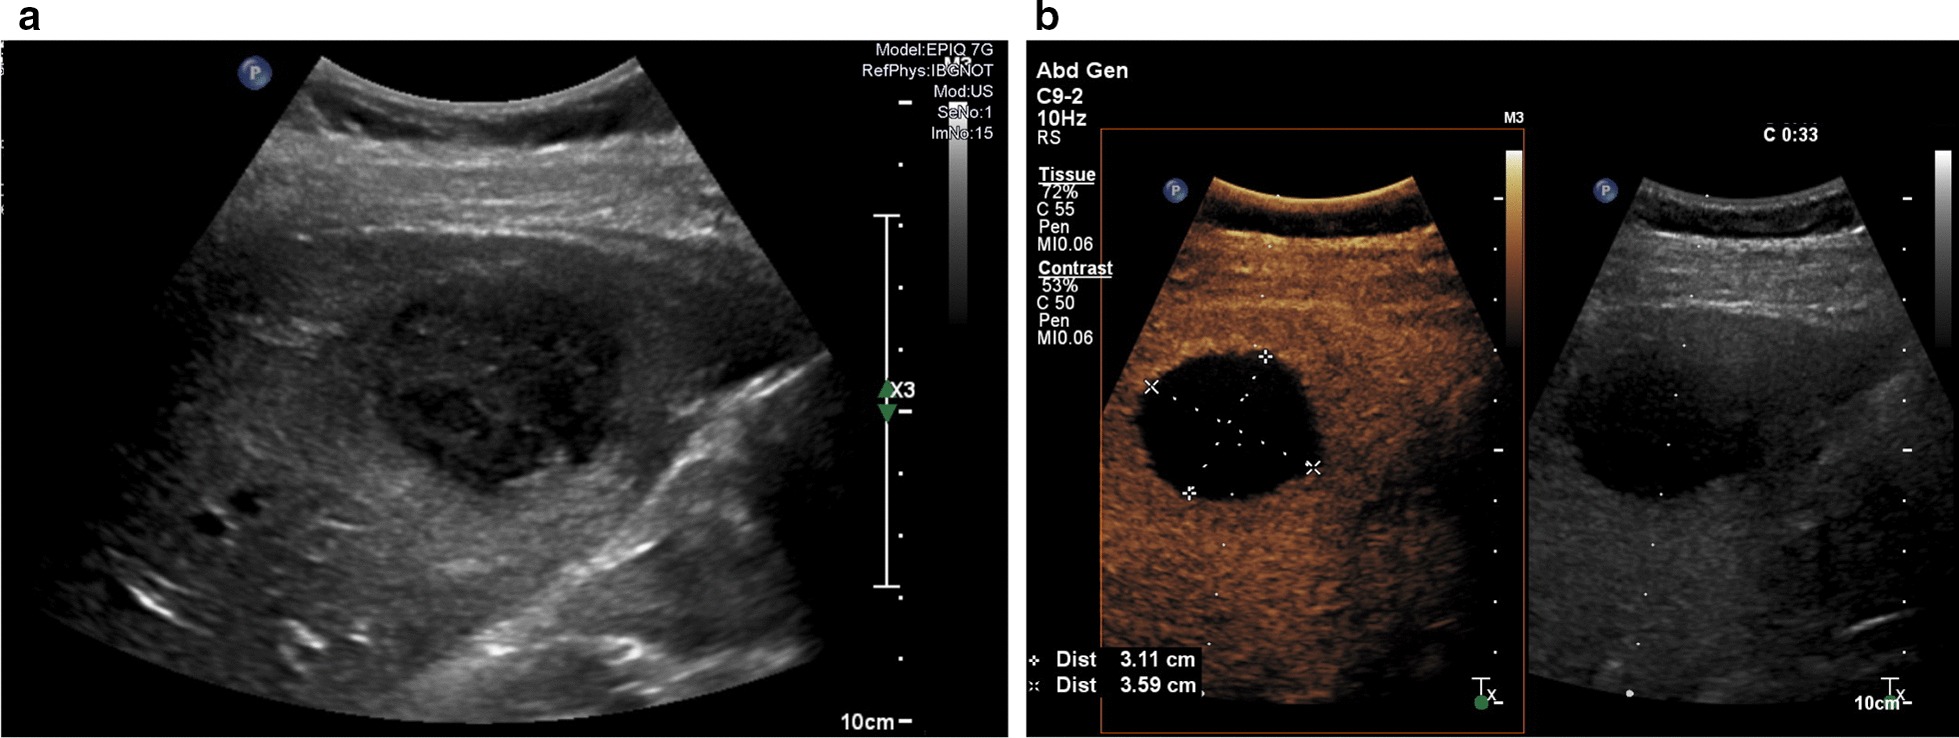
a 2D abdominal ultrasound performed on the day of presentation shows a hypoechogenic, inhomogenous and approximately 4 × 5 cm large lesion in hepatic segment V. b Contrast-enhanced ultrasound of the liver reveals peripheral hypervascularization of the lesion in segment V without central uptake of contrast, a finding consistent with liver abscess
